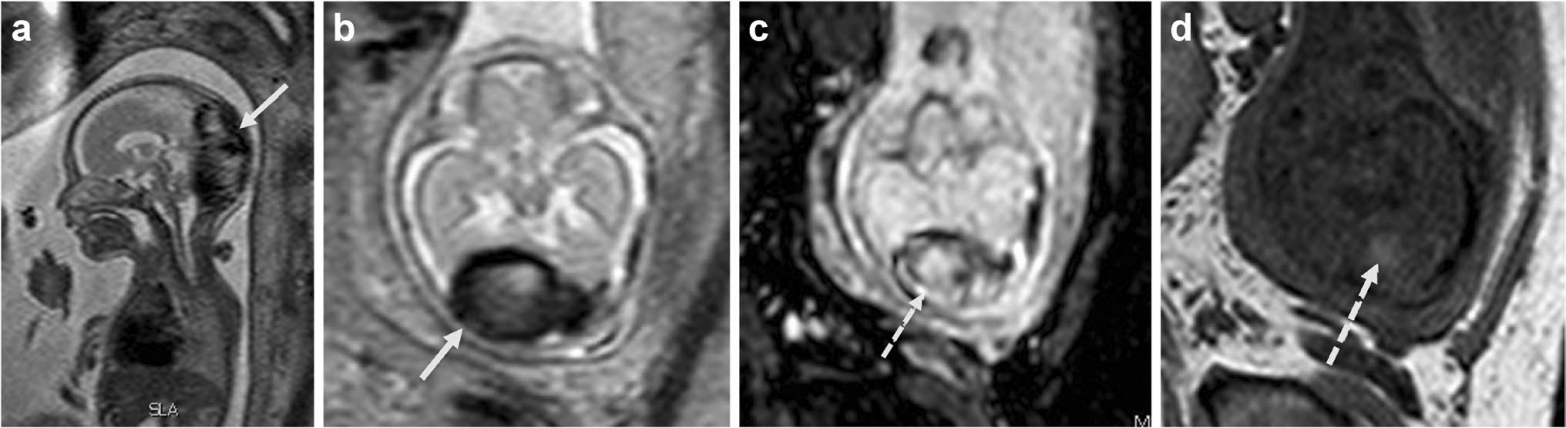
Dural arteriovenous malformation in a male fetus at 25 weeks gestational age whos had ventriculomegaly and an anechoic midline structure of the posterior fossa on prenatal ultrasound (not shown), for which magnetic resonance imaging was indicated. a, b Sagittal (a) and axial (b) T2-weighted images reveal a prominent extra-axial, heterogenous flow-void (*arrow*) at the posterior part of the cranium, in keeping with dural arteriovenous malformation. c, d On axial T2* (c) and T1-weighted (d) images, a round structure (*broken arrow*) is identified within the flow-void, suggesting a thrombus
